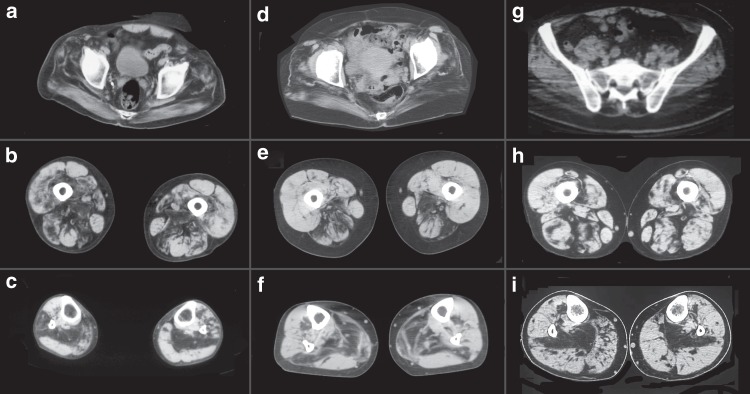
Muscle imaging in myoglobinopathy. Muscle CT scan from individuals F1, II: 7 (a–c); F4, II: 4 (d–f); and F5, II: 6 (g–i) at the pelvic (a, d and g), mid-thigh (b, e, and h), and mid-leg (c, f, and i). At the pelvis, there is involvement of the gluteus maximus, medius, and minimus. At mid-thigh, there is preferential involvement of the posterior compartment, specially, of the adductor magnus, biceps femoris, and semimembranosus. At the mid-leg, the soleus is the first and most-affected muscle
